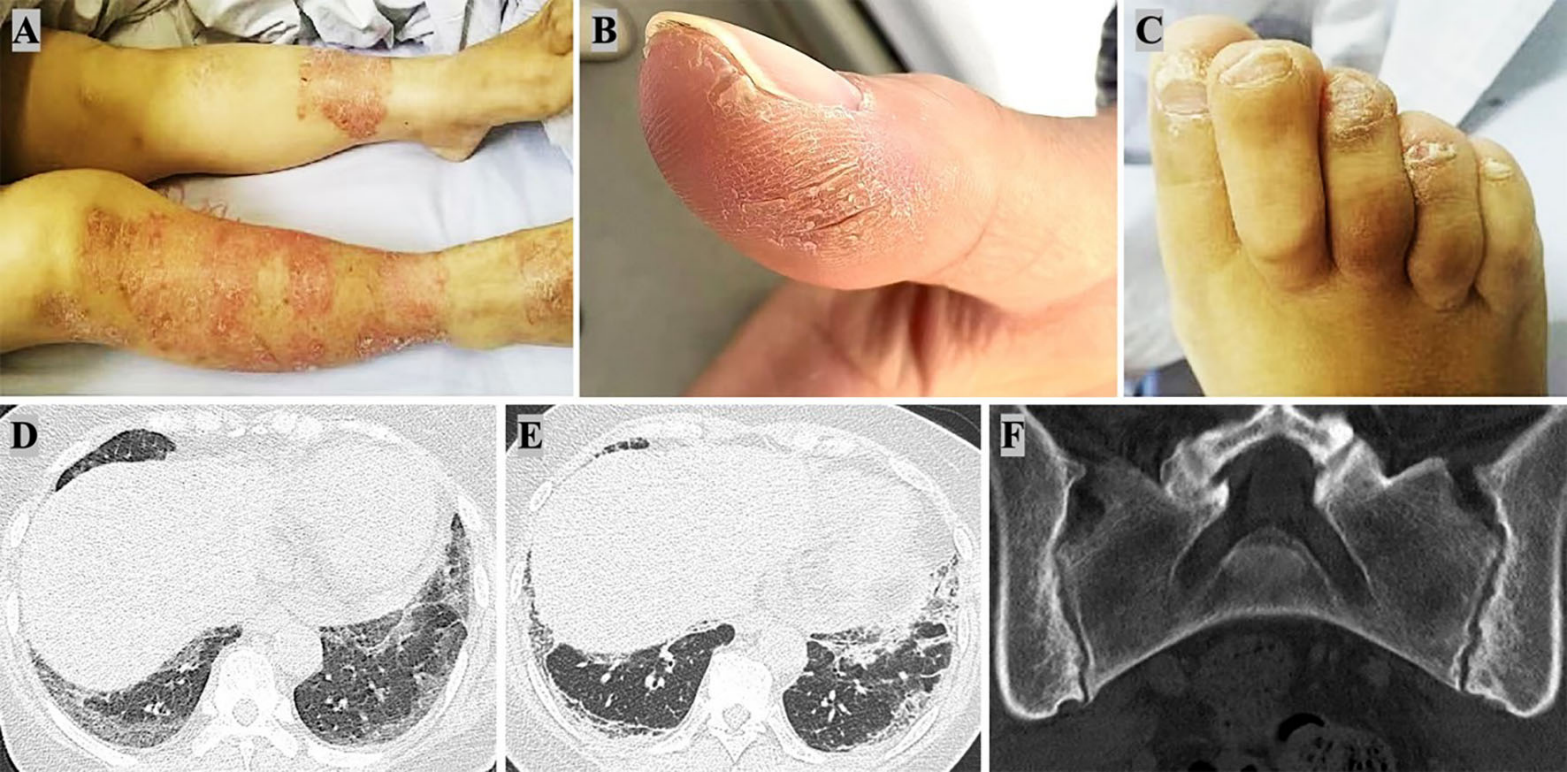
Clinical and Radiological Manifestations of Antisynthetase Syndrome in a Patient with Psoriatic Arthritis. (A) Scaly, erythematous plaques with well-demarcated borders distributed on the lower extremities, characteristic of psoriasis. (B) Hyperkeratosis and fissuring of the thumb, consistent with mechanic’s hands, a hallmark feature of antisynthetase syndrome. (C) Thickened, dystrophic toenails with onychodystrophy and dactylitis, commonly seen in psoriatic arthritis (PsA). (D) High-resolution computed tomography (HRCT) scans showing interstitial lung disease with a nonspecific interstitial pneumonia (NSIP) pattern, which is frequently associated with antisynthetase syndrome (on admission). (E) Interstitial lung disease exacerbated because of inadequate treatment. (F) Computed tomography (CT) scan of the sacroiliac joints showing mild bilateral sacroiliitis, which can occur in psoriatic arthritis.
